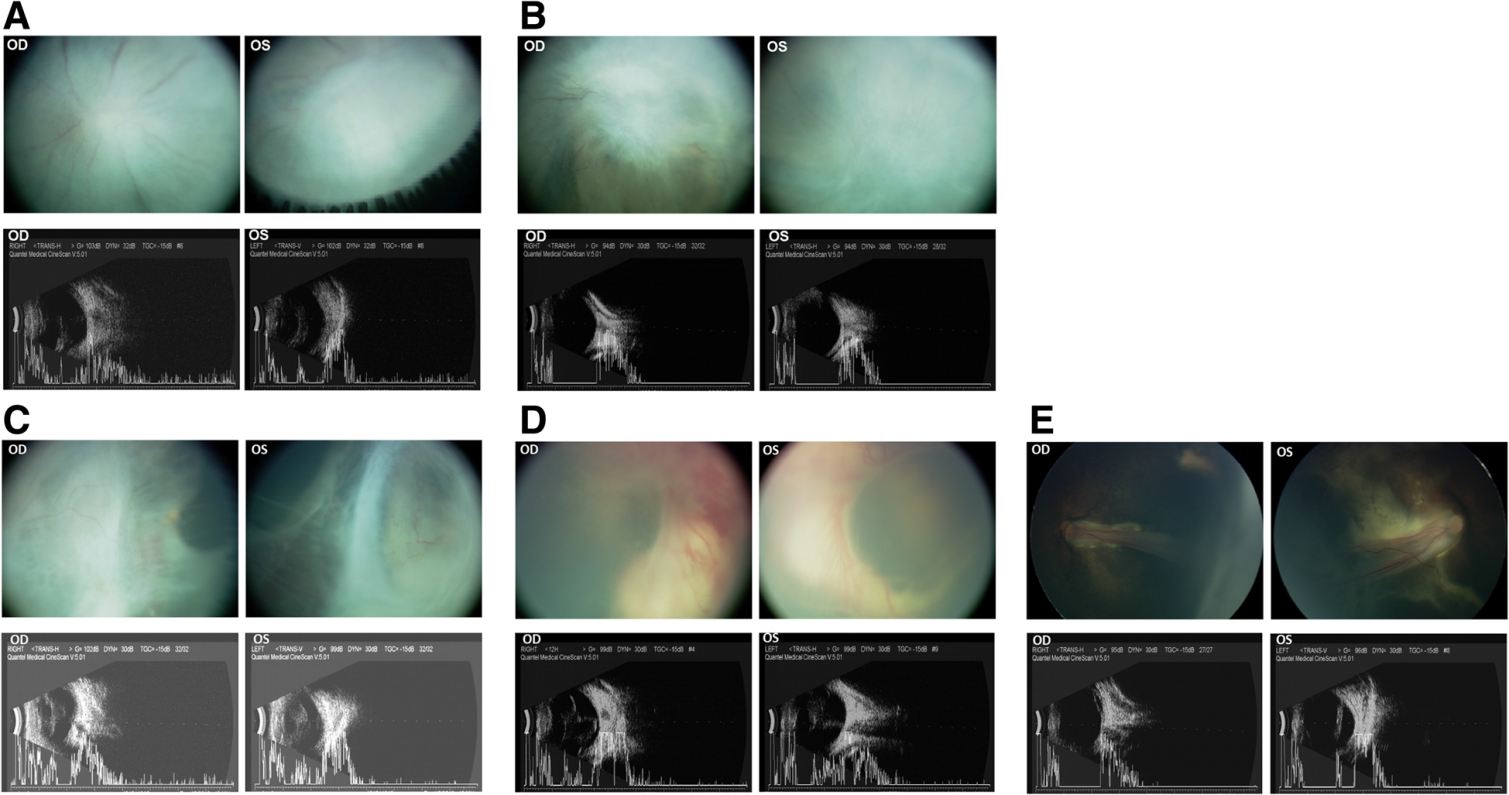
Representative images of retinal detachment (RD) of advanced stages of retinopathy of prematurity (ROP). a. A four-month-old boy infant from Inner Mongolia with 1600 g of birthweight. Top image, RD accompanied by peripheral fibrous traction to cilliary body in two eyes of one infant. Bottom image, the ultrasound of the corresponding RD with fibrosis membrane seen extending from peripheral retina to the edge of cilliary body, b. A ten-month-old boy infant from Gansu Province with 2100 g of birthweight. Top image, RD accompanied by a thick fibrous traction membrane in two eyes of one infant. Bottom image, the ultrasound of the corresponding RD with fibrosis membrane seen spreading through the whole retina, c. A three-month-old boy infant from Gansu Province with 1250 g of birthweight. Top image, RD accompanied by centripetal traction of neuro-sensory retina towards to optic discs along the major vascular arcade and formation of retinal fold. Bottom image, the ultrasound of the corresponding RD, d. A two-month-old girl infant from Gansu Province with 2000 g of birthweight. Top image, RD accompanied by fibrous membrane and retinal bleeding. Bottom image, the ultrasound of the corresponding RD, e. A six-month-old boy infant from Gansu Province with 1500 g of birthweight. Top image, retina rolled-up as a stalk anteriorly to just behind the lens. Bottom image, the ultrasound of the corresponding RD
